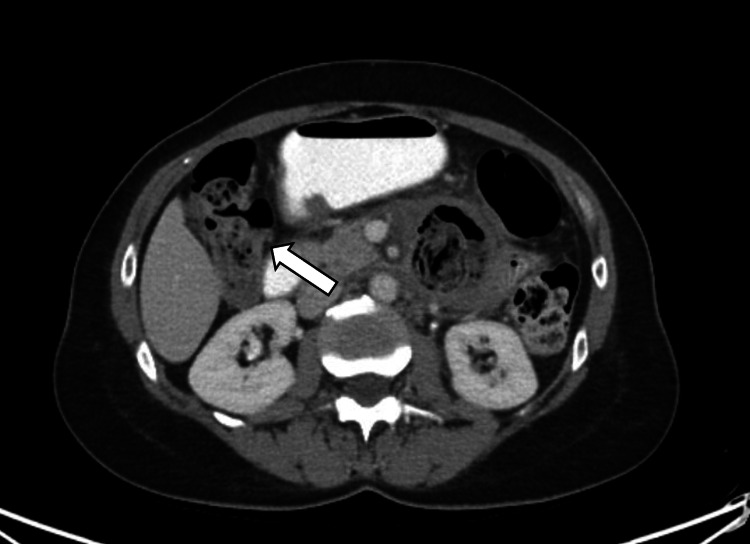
Axial contrast-enhanced CT image demonstrating a suspected duodenal diverticulum containing intraluminal material (arrow), with surrounding inflammatory changes suggestive of a contained perforation.
